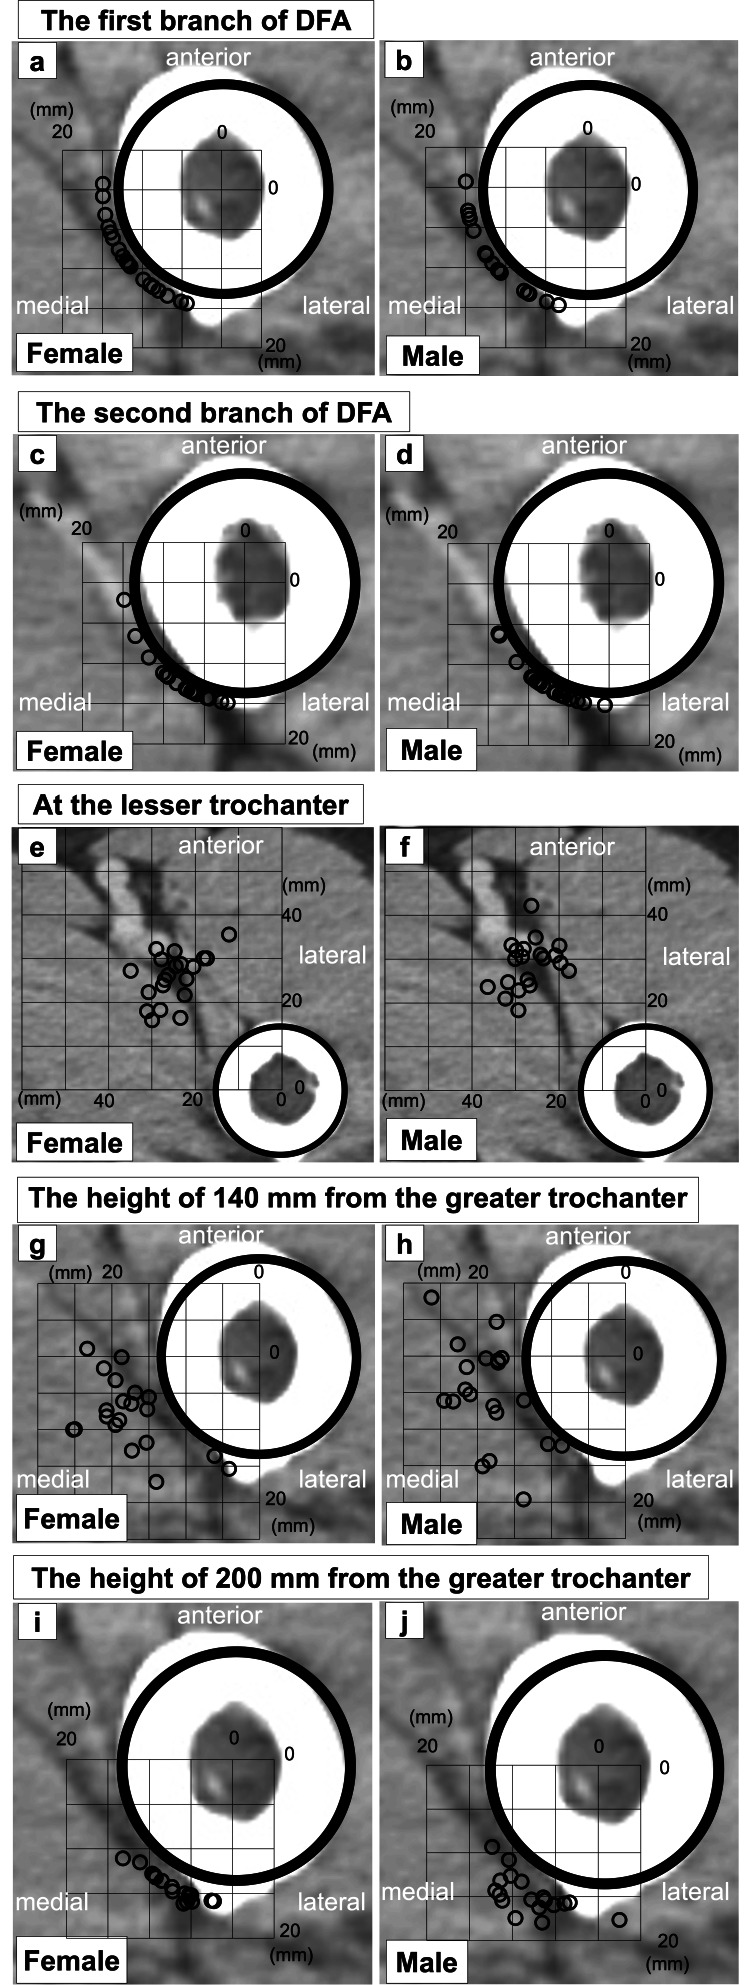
Distribution map of the deep femoral artery (DFA). Circles indicate the locations of the DFA on axial computed tomography images, reflecting positional variance among participants.
